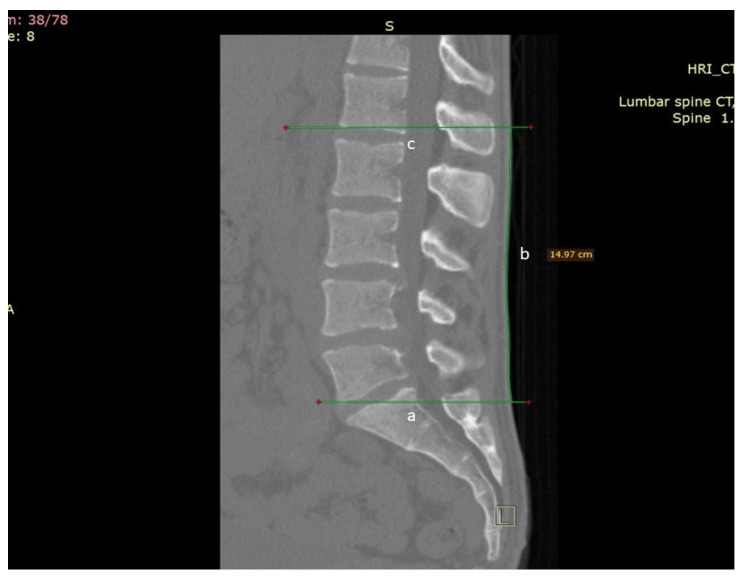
Actual lumbar (L1-S1) motion segments length measurement on CT. A line was stretched from the mid-endplate of S1 to the skin surface (marked as a), and a parallel line was extended from the mid-endplate of L1 to the skin surface (marked as c). The distance between both lines was measured on the skin surface (marked as b).
